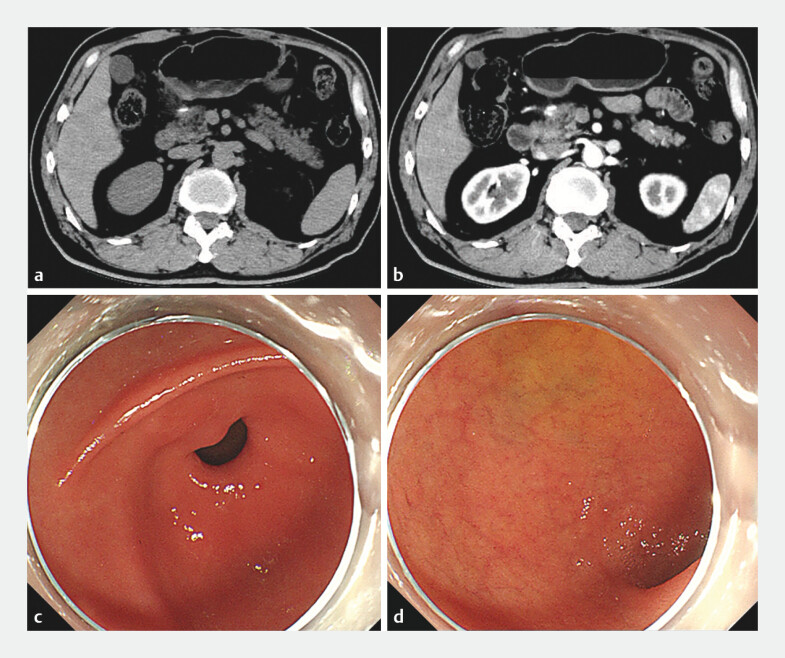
Abdominal computed tomography (CT) and gastroscopy images before the endoscopic
